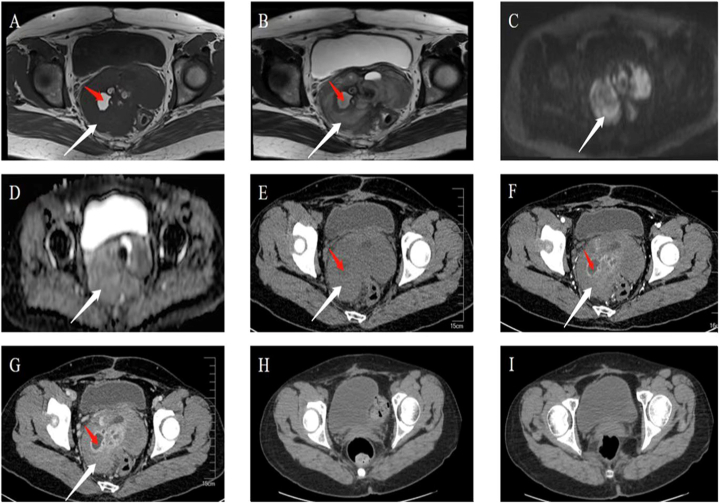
A-D. MRI scans showed multiple shadows of soft tissues (white arrow) with uneven signals and confluent intensity on T1WI (hypointensity, A), T2WI (mixed hyperintensity, B), DWI (hyperintensity, C), and ADC (hypointensity, D). The signal was uneven in the lesion, and multiple hyperintense lesions on T1WI and mixed hypointense lesions on T2WI were seen (red arrow). The lesion had a blurred margin with the adjacent rectum and sigmoid colon. E-G. Plain computed tomography (CT) (E), the CT arterial phase (F), and the venous phase (G) showed irregular lobular mass shadows behind the bladder (white arrow), in which the intensity was uneven and cystic regions were detectable (red arrow). The contrast-enhanced CT showed inhomogeneous enhancement in the lesion with local ring enhancement. Enhancement was not detectable in the cystic regions. The rectum and sigmoid colon were partially surrounded, and the boundary with the posterior wall of the bladder was blurred. Postoperative CT review: There was no abnormal density shadow in the pelvic cavity (H–I).
